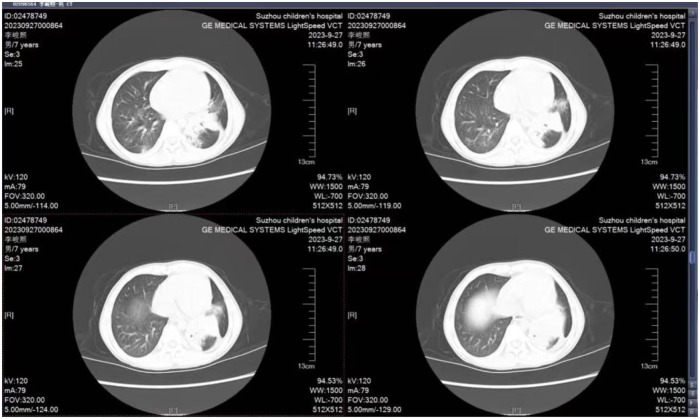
Chest CT (2023-09-27): slice and cable high-density shadows in both lungs, with the left lower lobe.
